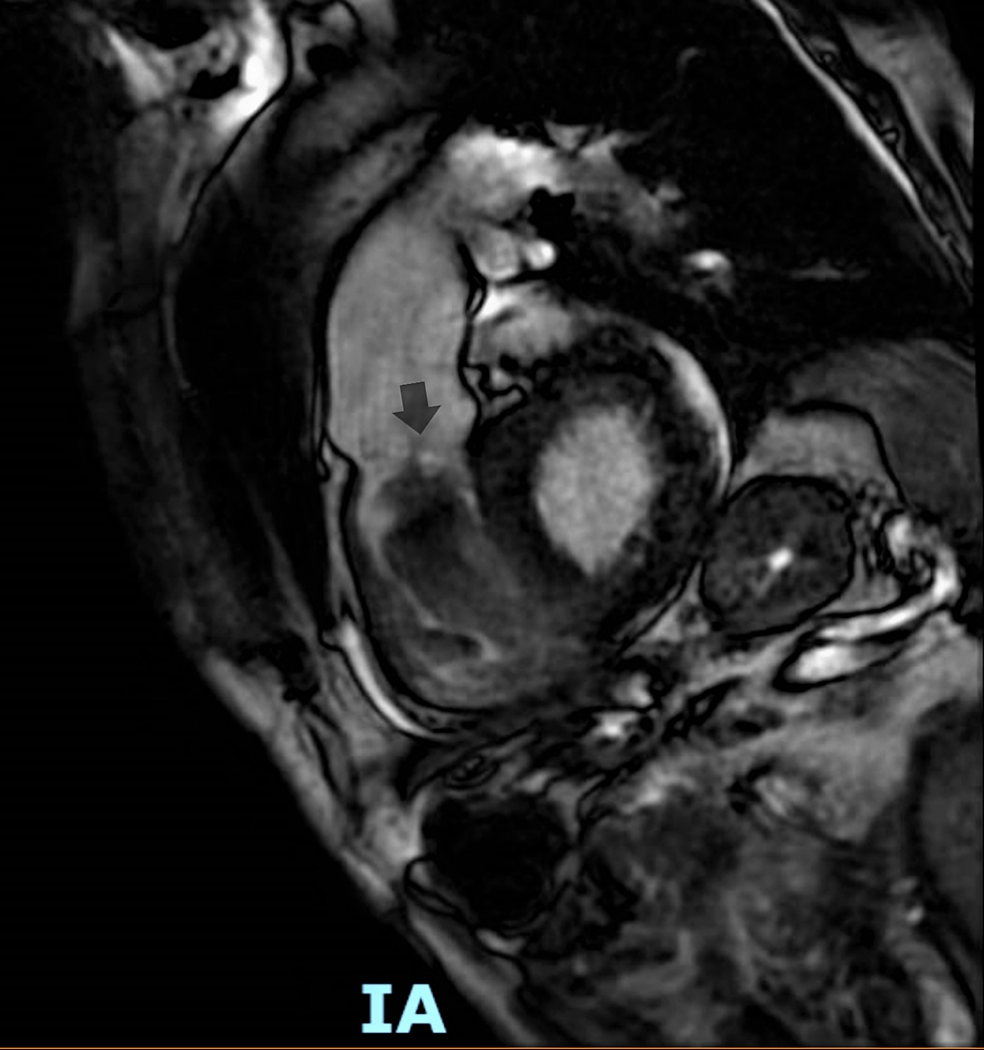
Cardiac MRI showing a large right ventricular mass attached to the endocardium on the RV septal aspect and the anterior aspect of the RVOT (the arrow is pointing toward the intra-cardiac tumor) RV, right ventricular; RVOT, RV outflow tract
